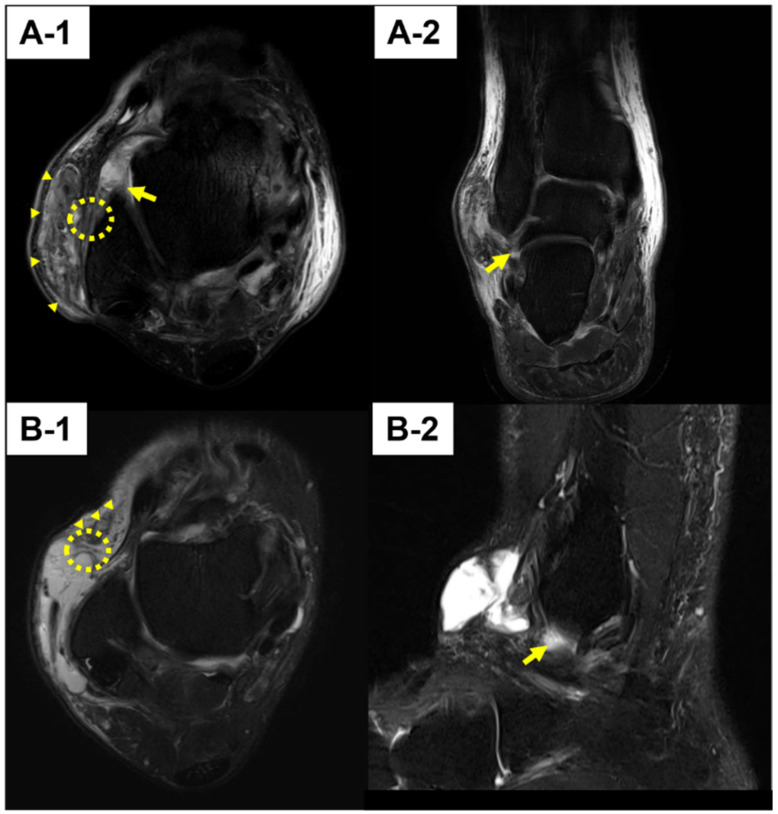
MRI findings. (A-1) Bursitis, located on the lateral malleolar area (Tailless arrows), connected with the synovial fistula (Dotted circle), along with chronic anterior talofibular ligament rupture (Arrow). (A-2) Along with lateral malleolar bursitis connected with synovial fistula, chronic rupture of calcaneofibular ligament (Arrow). (B-1) Bursitis located on the premalleolar area (Tailless arrows), connected with the synovial fistula (dotted circle). (B-2) Along with premalleolar bursitis connected with synovial fistula, chronic rupture of anterior talofibular ligament (Arrow).
